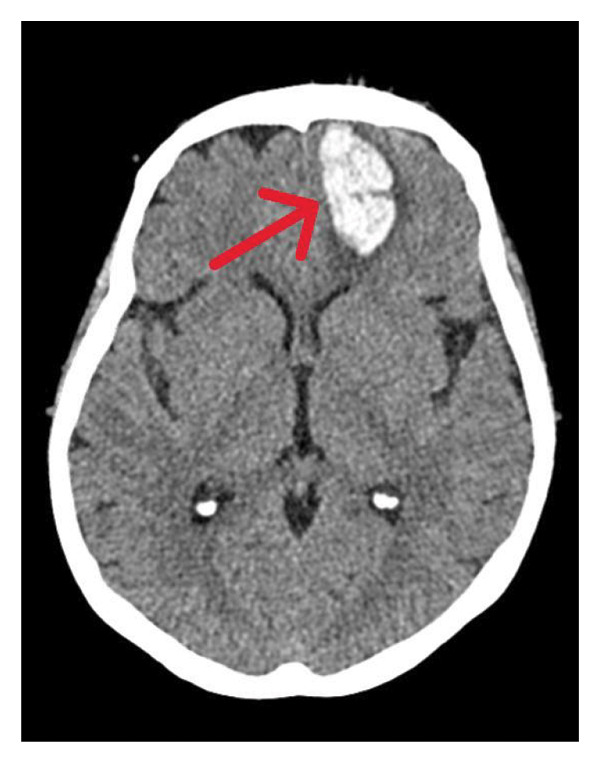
Noncontrast brain CT showing a large left frontobasal intracerebral hemorrhage, measuring approximately 2 × 3.8 × 4.7 cm, with no midline shift or significant ventricular compression.
